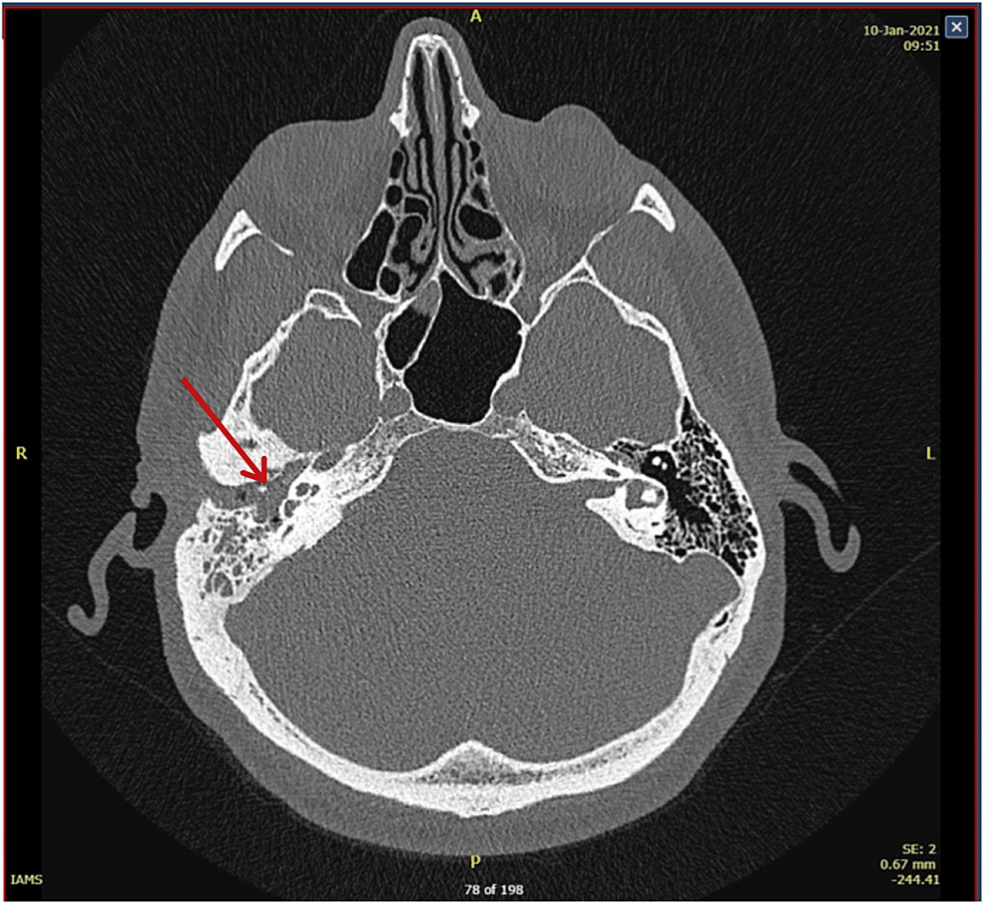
CT of the temporal bones. A CT scan of the temporal bones revealed opacification in the right middle ear, highlighted by an arrow, suggesting erosion of the malleus and incus.
